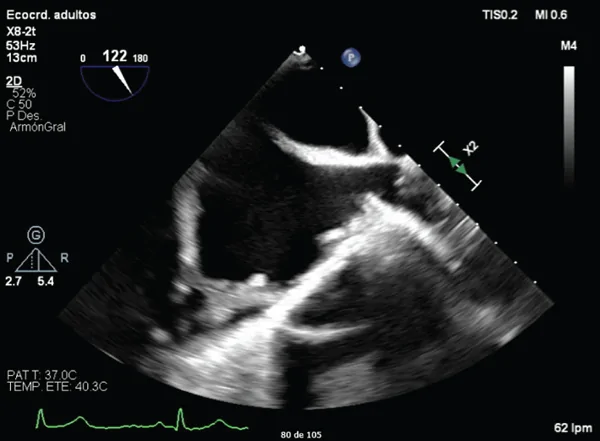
Bicaval transesophageal echocardiography view at 122°. A linear hyperrefringent structure is visualized outside the right atrial cavity, extending into the right ventricle and consistent with cement embolus. This finding is associated with a small pericardial effusion, without initial signs of cardiac tamponade.
